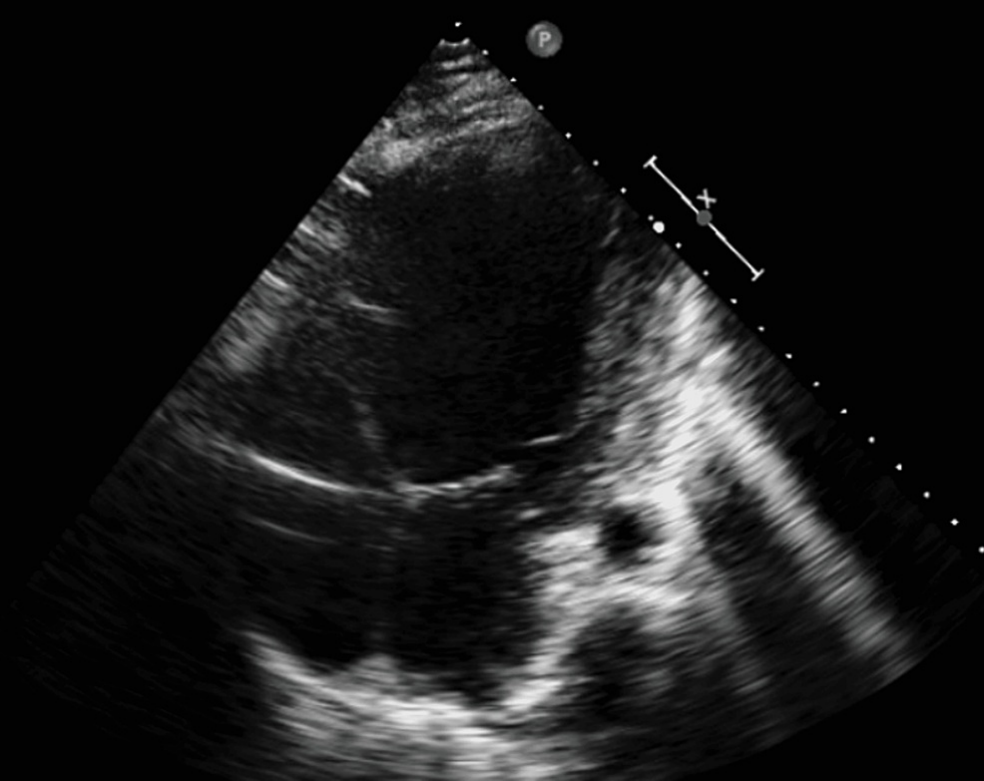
Two-dimensional echocardiogram This view of echo shows that there is a left heart strain signifying a severe left ventricular systolic dysfunction. Echo also reveals an ejection fraction of 10-15% demonstrating that there is an acute decompensation of heart failure.
